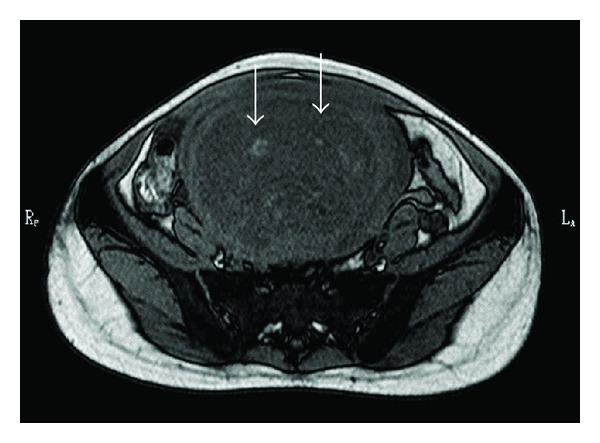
Magnetic resonance imaging of the uterine tumor showing a small area of high-signal intensity (arrows) on a T1-weighted image.
